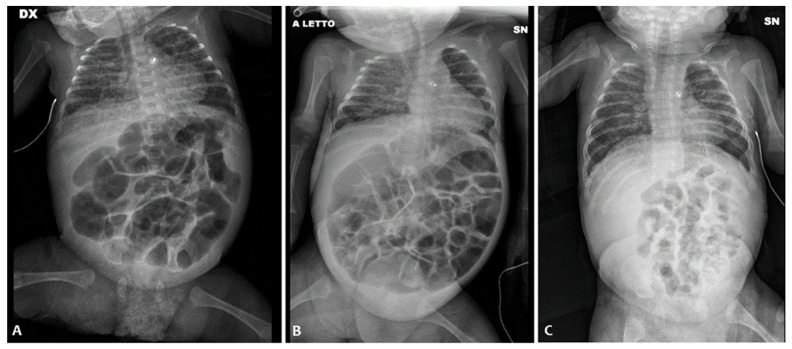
Abdominal X-ray of patient P2 on the 36th day of life (A); persistent abdominal distension on the 86th day of life (B); and abdominal X-ray after the NAC treatment (C).
